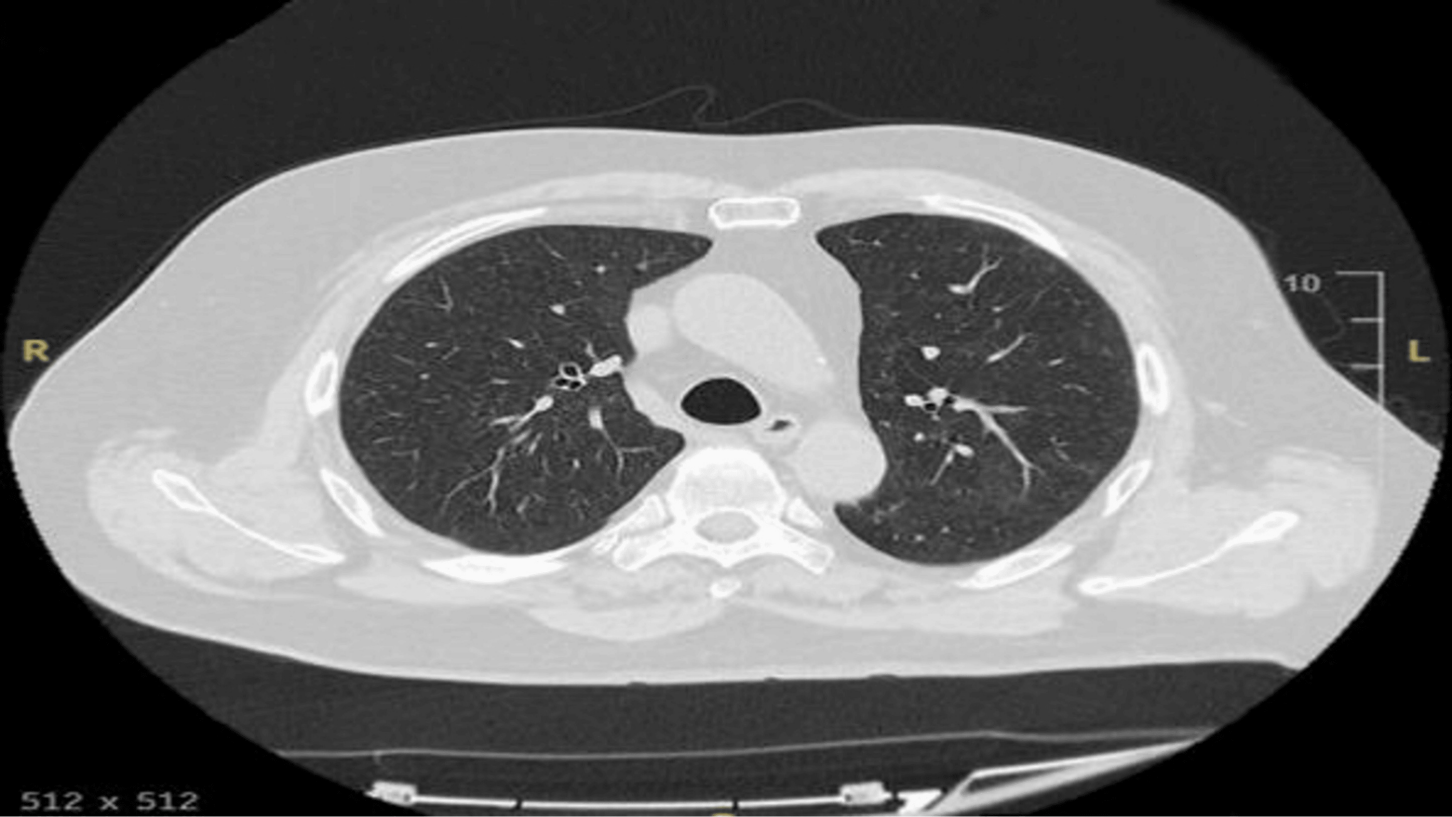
CT chest from one year ago: cancer surveillance follow-up The patient had a CT chest performed one year prior as part of a cancer surveillance follow-up, which was reported as normal. CT, computed tomography
